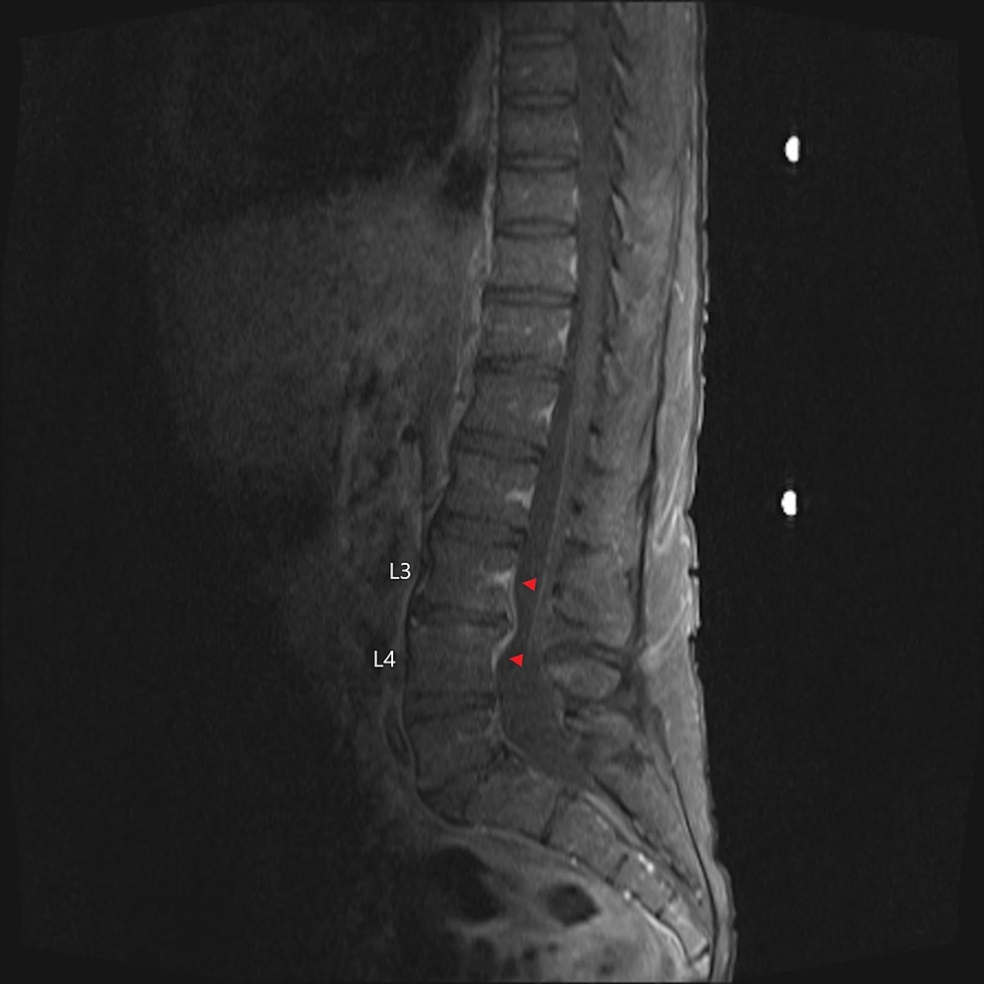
Pre-treatment T1 fat-suppressed post-gadolinium MRI sagittal view showing signal enhancement of the L3/L4 vertebral bodies (red arrowheads). MRI: magnetic resonance imaging
